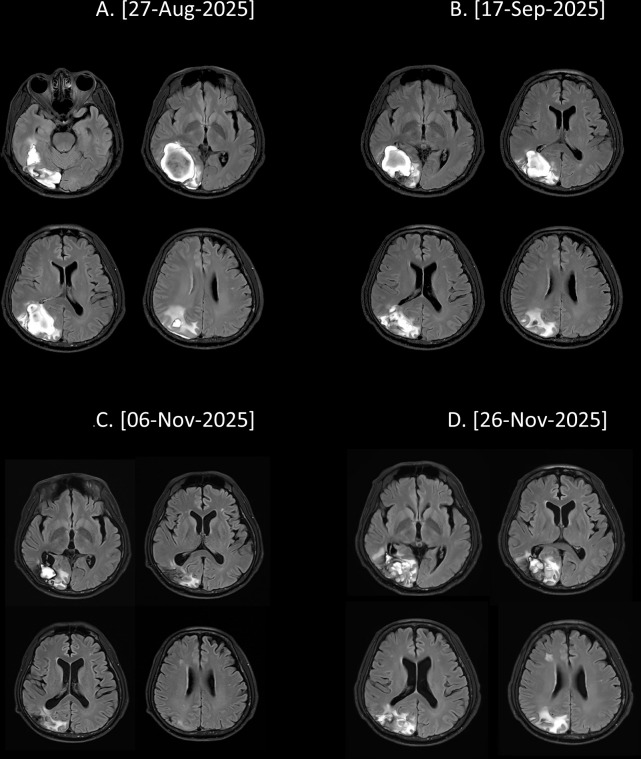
Serial brain MRI findings of intracerebral hemorrhage during lenvatinib therapy. (A) Contrast-enhanced brain MRI performed on August 27, 2025, demonstrated an approximately 6-cm acute-to-subacute lobar intracerebral hemorrhage involving the right parieto–occipito–temporal lobes, accompanied by surrounding vasogenic edema and irregular peripheral enhancement. (B) Follow-up brain MRI performed on September 17, 2025, showed a slight decrease in the size of the hemorrhagic lesion in the right temporo-occipital lobe, while the peripheral enhancing portion remained unchanged. Differential diagnoses included hemorrhagic metastasis and cavernous malformation with multistage hemorrhage. (C) Follow-up MRI obtained on November 6, 2025, demonstrated marked reduction in the size of the hemorrhagic lesion with decreased peripheral enhancement, reduced vasogenic edema, and resolving gliosis, suggestive of resolving multistage intracerebral hemorrhage. (D) Follow-up brain MRI performed on November 26, 2025, revealed interval aggravation of the previously identified intracerebral hemorrhage in the right temporo-occipital lobe during continued lenvatinib therapy.
